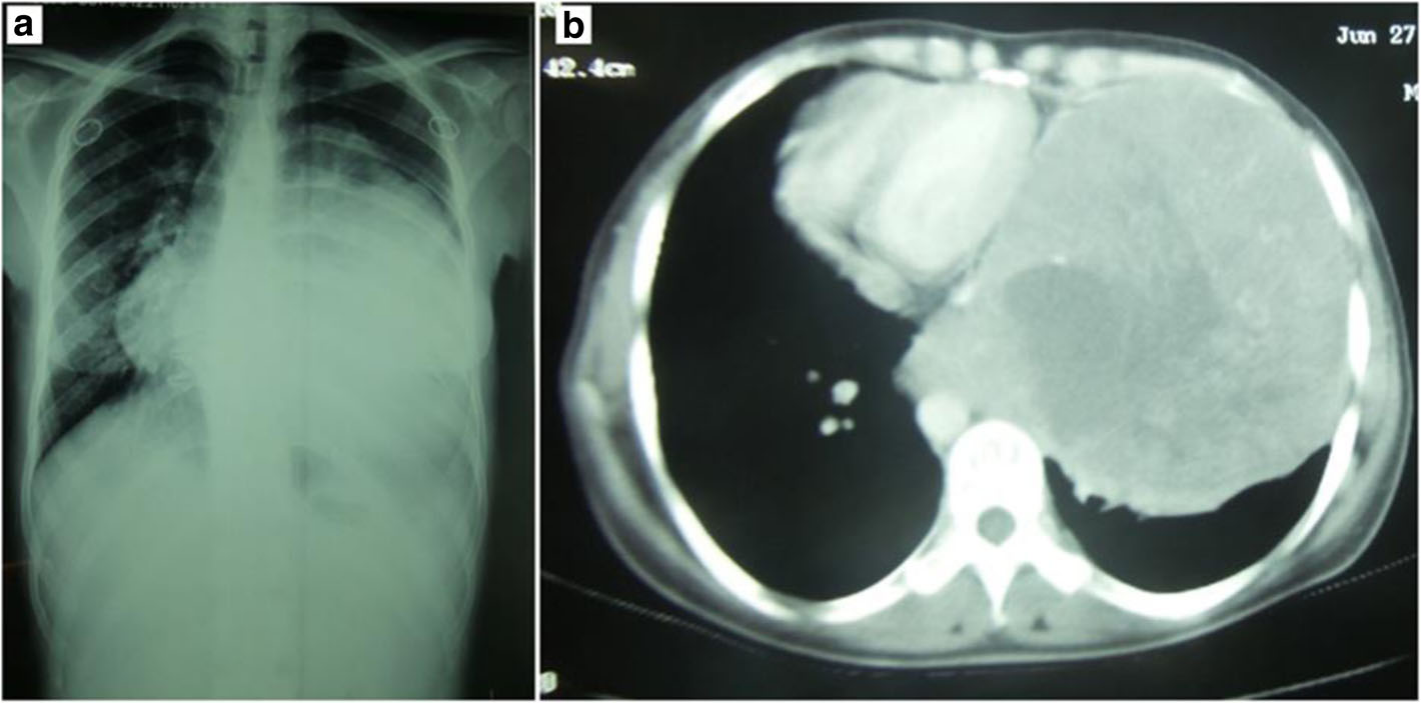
A chest X-ray shows a homogenous left-sided opacity occupying almost the entire left hemithorax, with non-visible left diaphragmatic dome (a). A chest computed tomography scan reveals an enormous mediastinal mass with a central area of necrosis and compression of mediastinal structures towards the right side (b)
